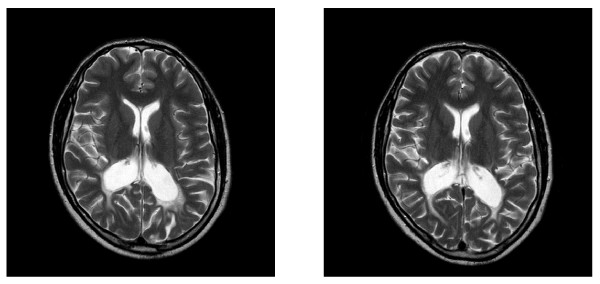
Brain MRI scans of the AMN patient: T2 weighted one month before DHA treatment began (September 2008) (left) and T2 weighted six months after DHA treatment began (March 2009) (right).
